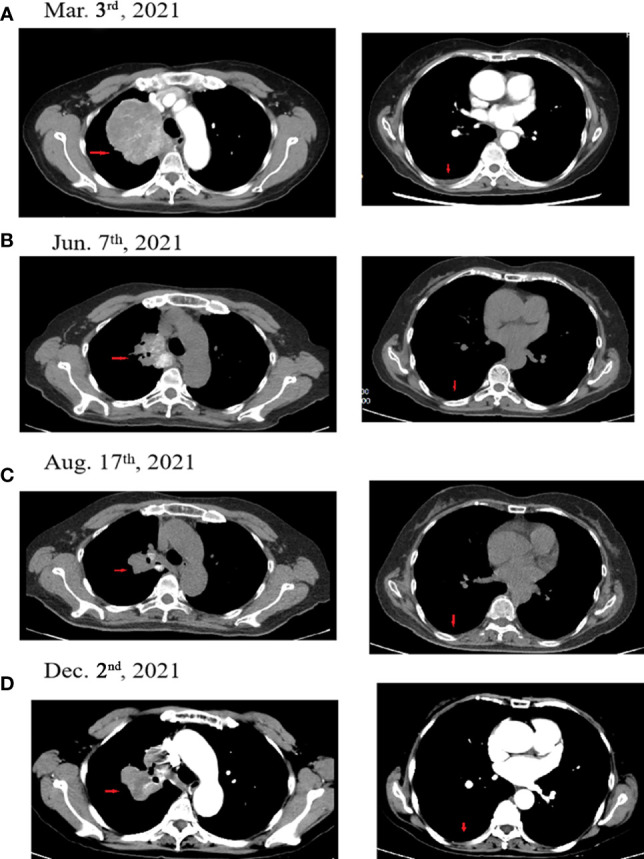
Computed tomographic (CT) images for the patient during the treatment course. (A) The patient experienced progressive disease during icotinib therapy (March 3, 2021). (B) The patient achieved PR following icotinib plus crizotinib therapy (June 7, 2021). (C) The patient achieved PR following icotinib plus crizotinib therapy (August 17, 2021). (D) The patient achieved SD following icotinib plus crizotinib therapy (December 2, 2021). Red arrows indicate the tumor.
